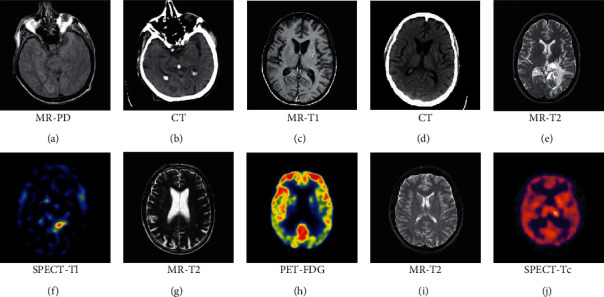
Five sets of multimodal medical images for the experiment. (a) MR-PD. (b) CT. (c) MR-T1. (d) CT. (e) MR-T2. (f) SPECT-Tl. (g) MR-T2. (h) PET-FDG. (i) MR-T2. (j) SPECT-Tc.
